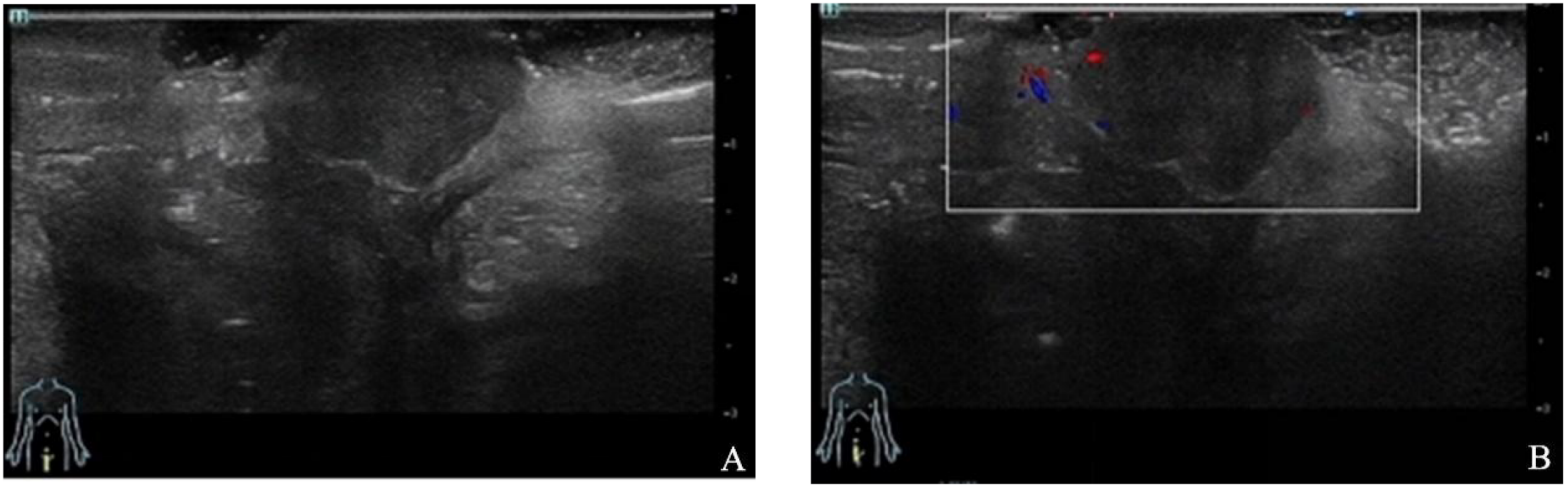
Ultrasound revealed a hypoechoic nodule with clear boundaries in the subcutaneous tissue of the right vulva.
